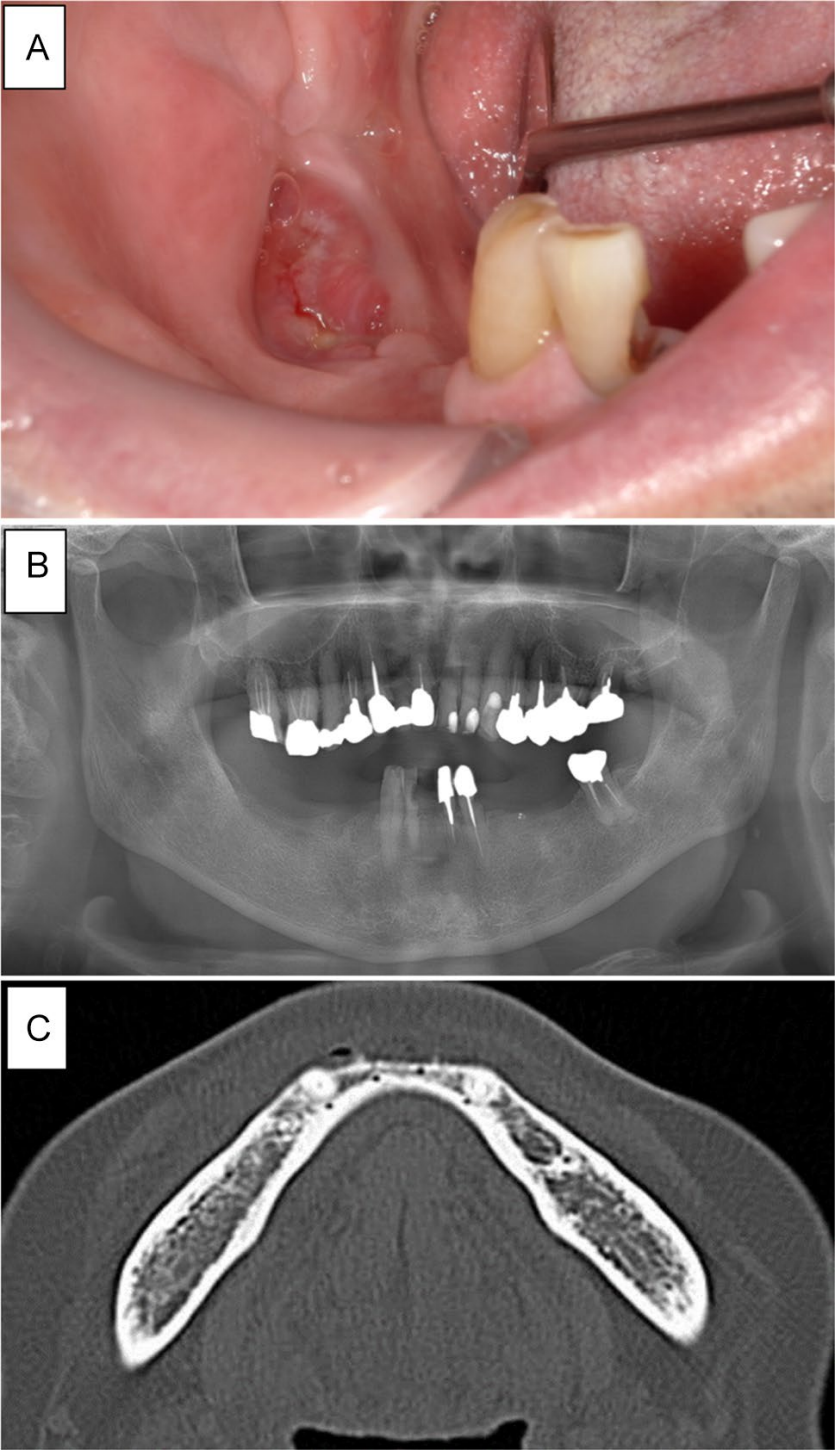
Intraoral and radiographic findings at the initial visit in case 1. (A) Exposure of the alveolar bone and redness of the surrounding gingiva are observed. (B) Panoramic radiograph showing no abnormal findings except for mild smooth bone resorption on the exposed bone surface. (C) Computed tomography showing no abnormal findings in the right mandible’s cortical bone or bone marrow.
